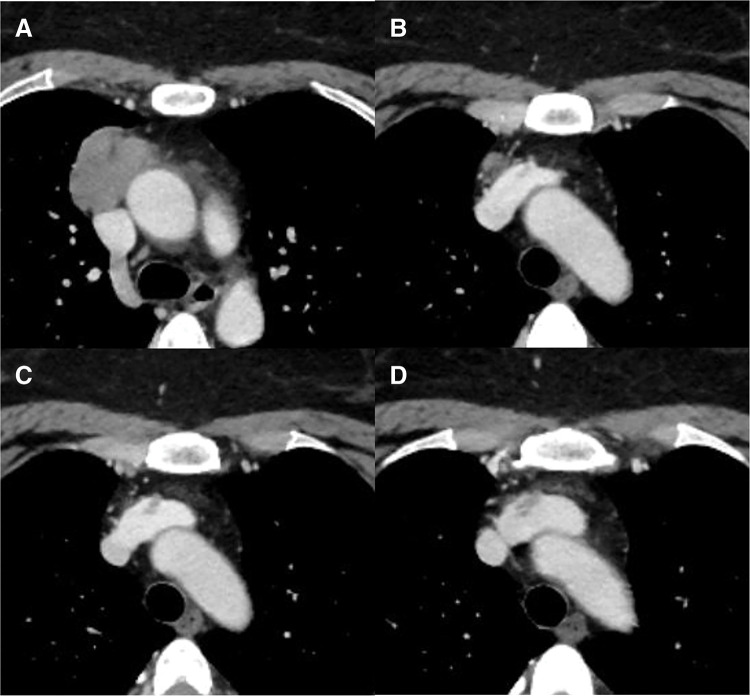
Preoperative imaging of the lesion. (A) Chest computed tomography (CT) displaying a mass (6.3 × 4.1 × 3.1 cm) in the anterior mediastinum. (B) The superior margin of the tumor. (C) A filling defect was observed in the left brachiocephalic vein. (D) A filling defect was also observed in the left brachiocephalic vein on follow-up CT.
